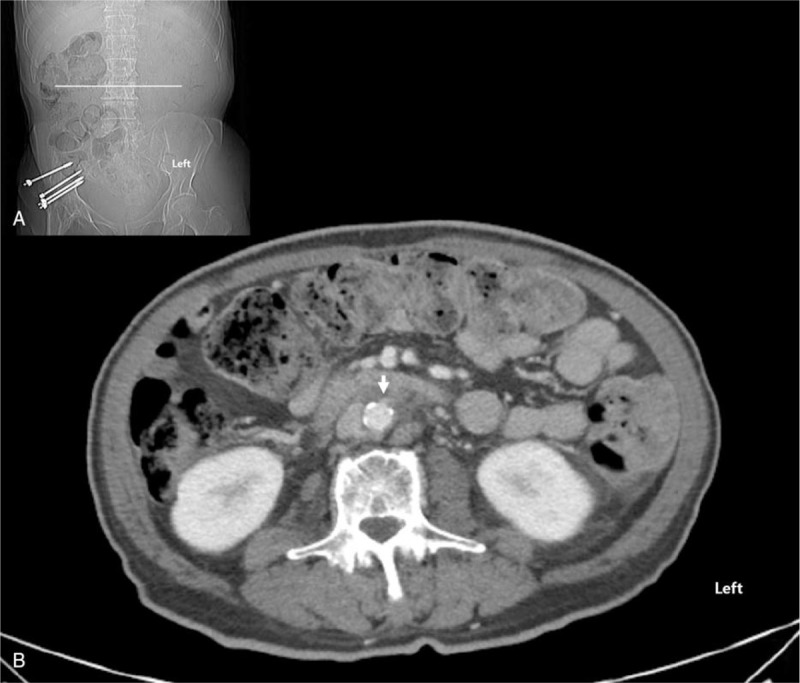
(A) Computed tomography (CT) scout view. (B) Axial CT image shows the inferior mesenteric artery (arrow) at L3 level.
